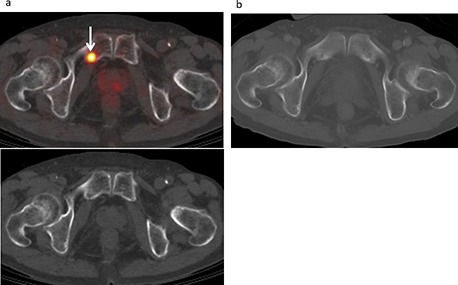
Bone metastasis developed in a 72-year-old male treated for malignant pleural mesothelioma, including pleurectomy and decortication procedures, and chemotherapy. (a) FDG-PET/CT and CT findings showed moderate FDG uptake (SUVmax 5.14) in the right pubic bone (arrow), suggesting bone metastasis with a score of 4. (b) Contrast-enhanced CT (bone image) results showed no abnormal FDG uptake with a score of 1 (false negative).
